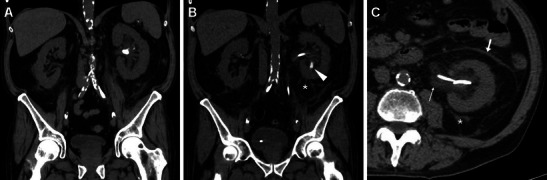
Usual early post-procedural imaging appearances following extracorporeal shock wave lithotripsy (ESWL) in a 51-year-old male with a 12-mm stone in the left renal pelvis detected by preoperative unenhanced multidetector CT (MDCT, A). Twenty-four hours after ESWL, repeat MDCT (B, C) was requested because persistent flank pain showed a ureteral stent in place, residual stone fragment in the lower pole calyx (*arrowhead in B*), minimal perirenal fluid (*) and ipsilateral fascial effusion (arrow in C), and thickened urothelium of the renal pelvis (*thin arrow in C*). Afterwards, the patient experienced an uneventful postoperative course
